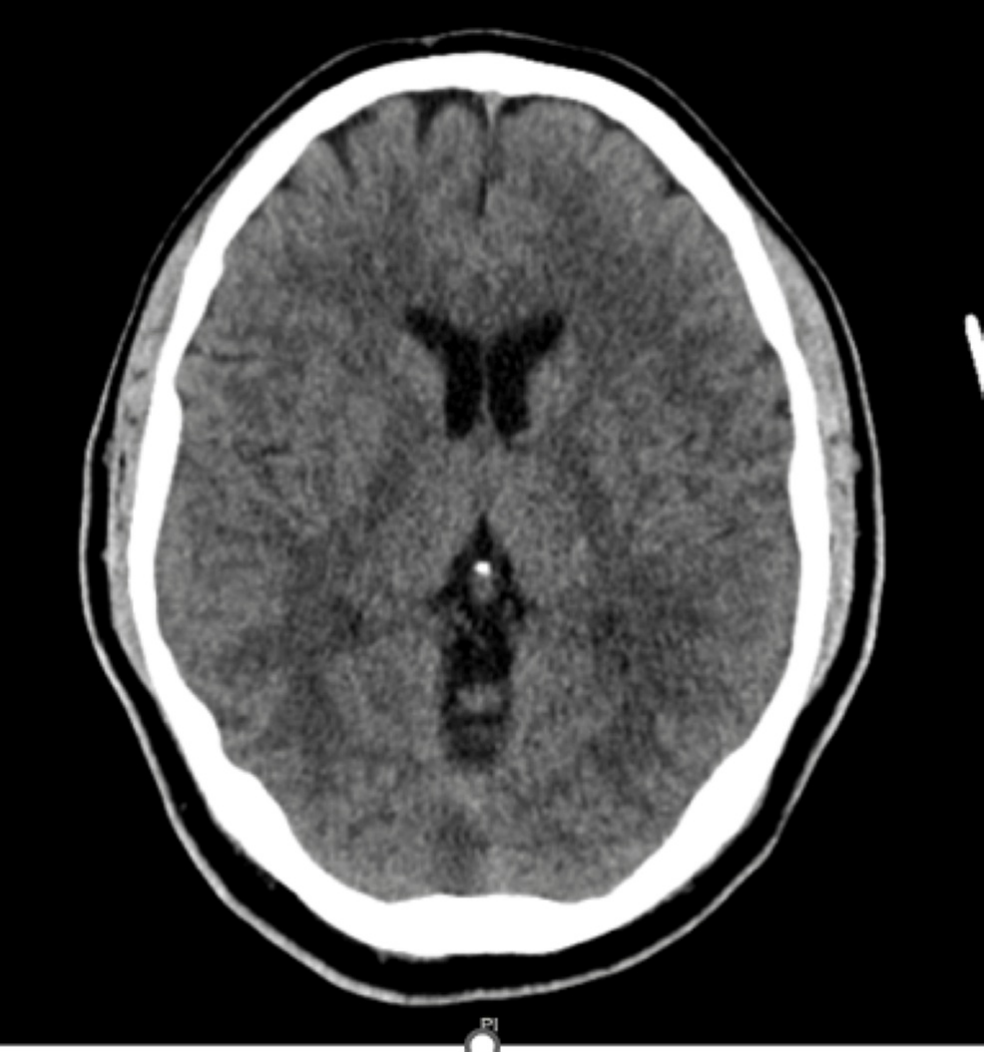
Computed tomography scan of the head without contrast: no acute abnormalities were noted
